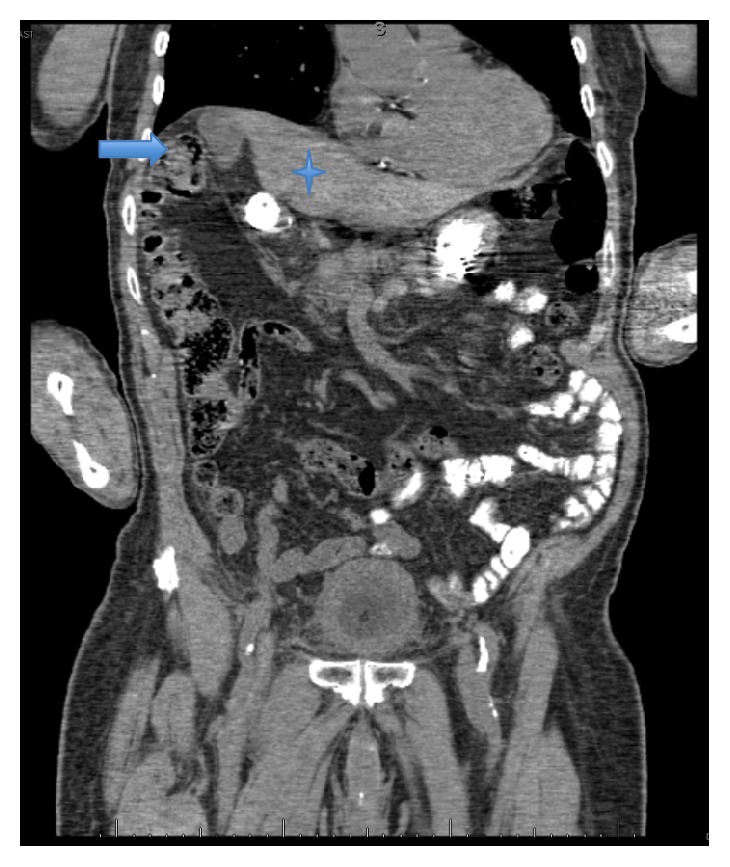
CT of the abdomen (coronal section) showing loops of colon (arrow) between the liver (asterisk) and the right hemidiaphragm.
